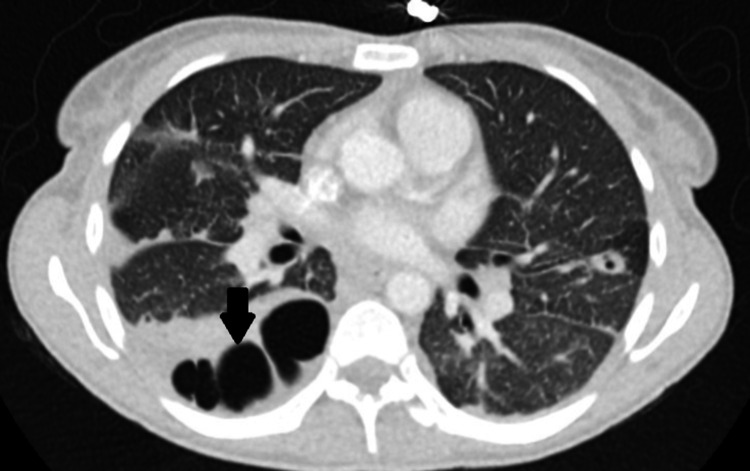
Chest CT with contrast. Chest CT scan demonstrating multiple lung cavitations (arrow).
